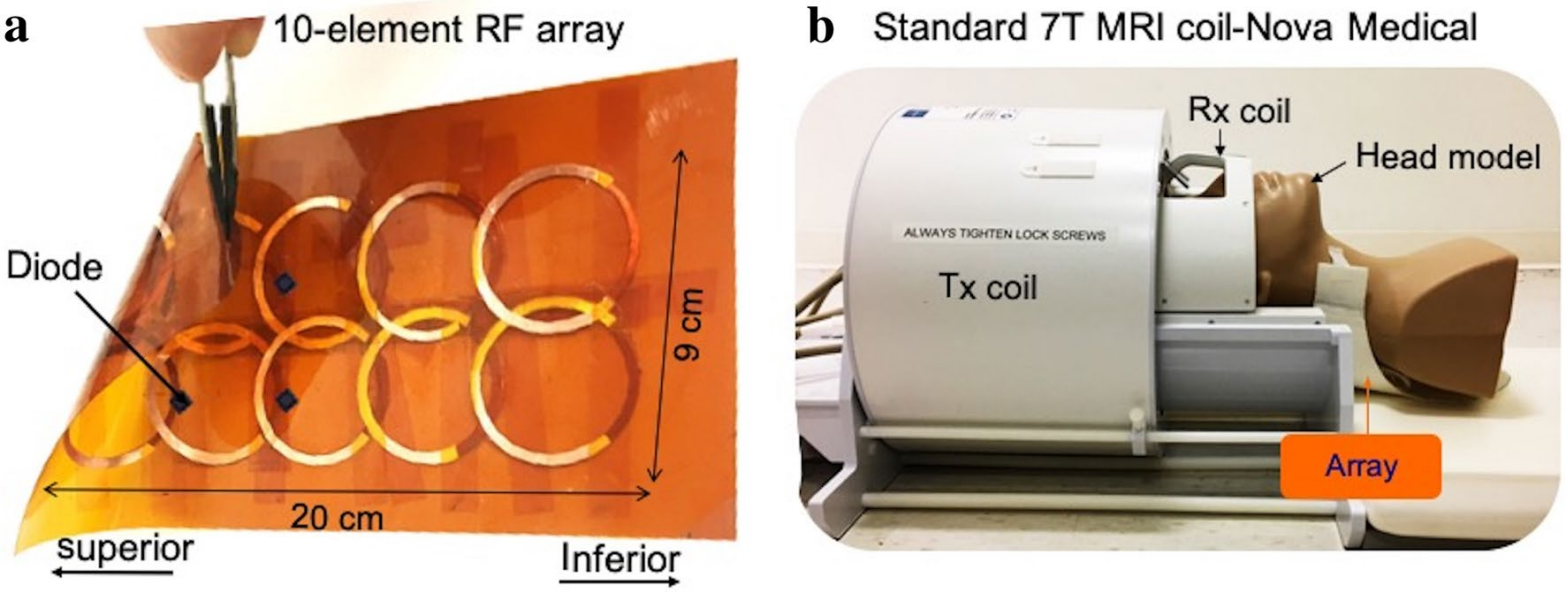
The prototyped RF array. (a) Prototype of the 10-element wireless passive RF resonator array constructed of 10 BCSRRs. Upon the substrate (Kapton), whose flexibility is shown by the instrument in the upper left, 10 SRRs were patterned on one side and 10 aligned but counter-oriented versions were applied to the other side. Critical overlapping distance (0.76D mm center-to-center) was used to reduce the inductive coupling between elements. Strongly coupled elements mainly in superior position were detuned using antiparallel cross diodes. (b) The picture of a standard head coil used in our MRI experiments and position of the array relative to the coil and a head model.
